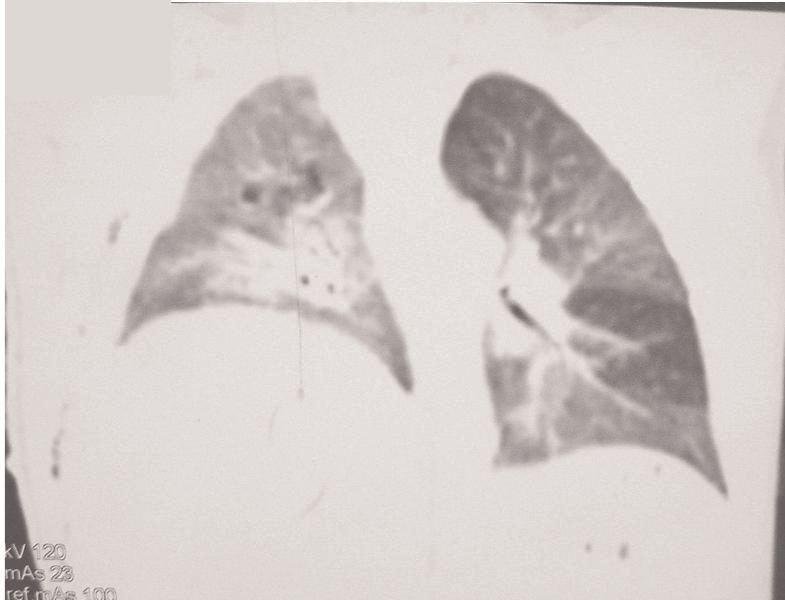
CT scan showing smooth outline elevated right hemidiaphragm.
